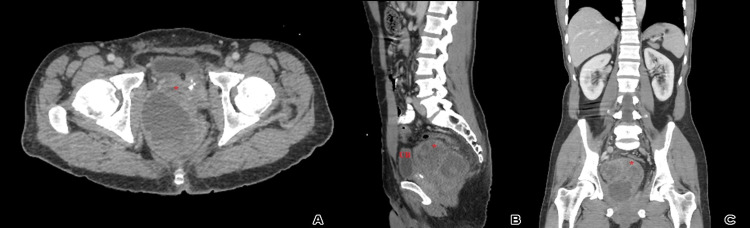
(A) Axial, (B) sagittal and (C) coronal pelvis CT scans with an IV contrast show a well-defined, lobulated, complex, heterogeneously enhanced soft tissue pelvis mass lesion (red asterisk), located between the urinary bladder (UB) and the sacrum with cystic changes, necrosis, and focal effect on the adjacent structures.
